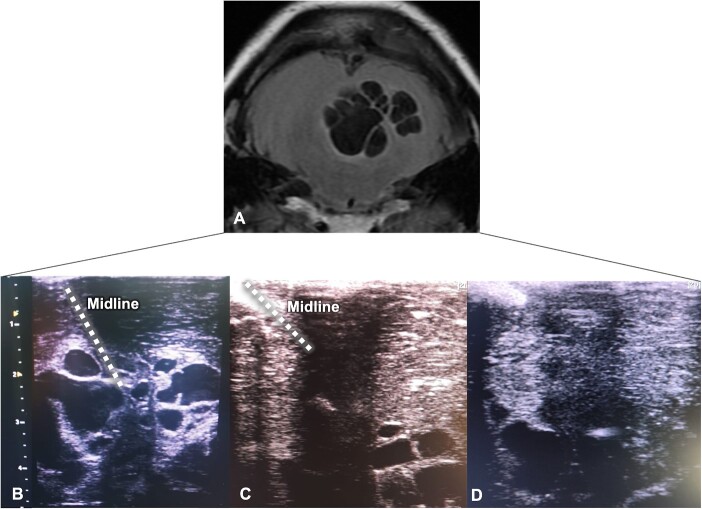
Intraoperative ultrasound-guided resection. (A) Cerebellar MRI is shown upside down for better correlation with the ultrasound view; parasitic cysts correspond to hypointense lesions; (B–D) Echographic views depicted the progression of ultra-sound guided resection with excision of the hypoechoic lesions from the superficial to deep planes.
